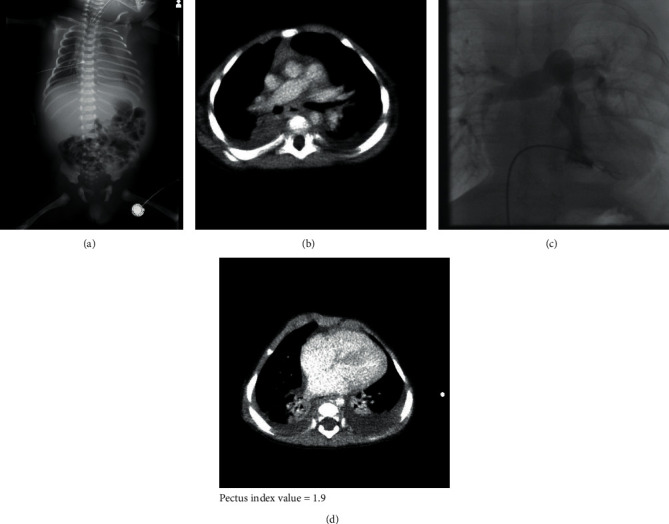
(a) Chest radiograph after birth. (b) Chest computed tomography (CT) and (c) right ventricular angiography. The arrows show supravalvular pulmonary stenosis. (d) Pectus index value calculated using CT-based imaging.
